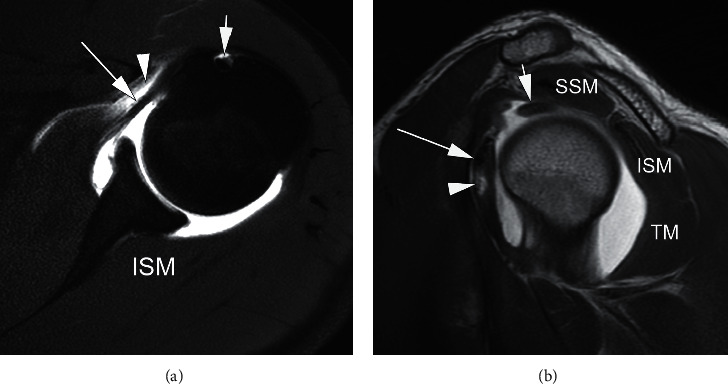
Normal MR arthrogram: axial T1-weighed FS (a) and sagittal T1-weighted (b) MRI show an intact subscapularis tendon (long arrow in a and b), long head of the biceps tendon (short arrow in a and b), supraspinatus muscle (SSM), infraspinatus muscle (ISM), and teres minor (TM). *Note.* In addition, there are some extra-articular contrast material deposition anterior to the subscapularis tendon (arrowhead in a) and minor contrast deposition within the musculotendinous junction of the subscapularis (arrowhead in b).
